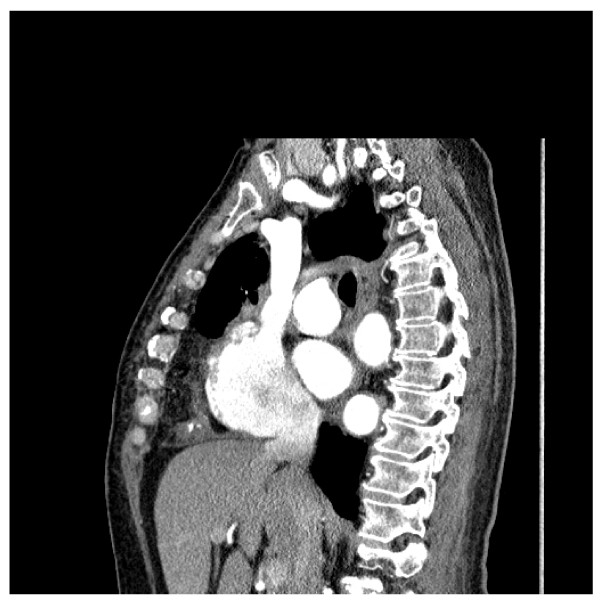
Follow-up CT-scan after one month with a decompressed right ventricle.
